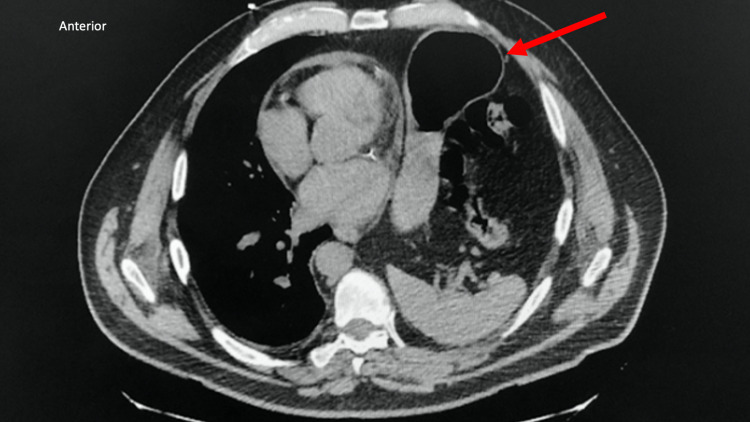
Thoracic CT Scan This transverse chest computerized tomography (CT) scan shows rightward mediastinal shift, coronary calcifications and stents, the elevation of the left hemidiaphragm, herniation of gastric contents into the thoracic cavity (arrow denotes the abnormal presence of abdominal contents, indicated by air-fluid levels, in the left thoracic cavity) with compressive atelectasis of the left lower lung, and lack of mediastinal adenopathy.
